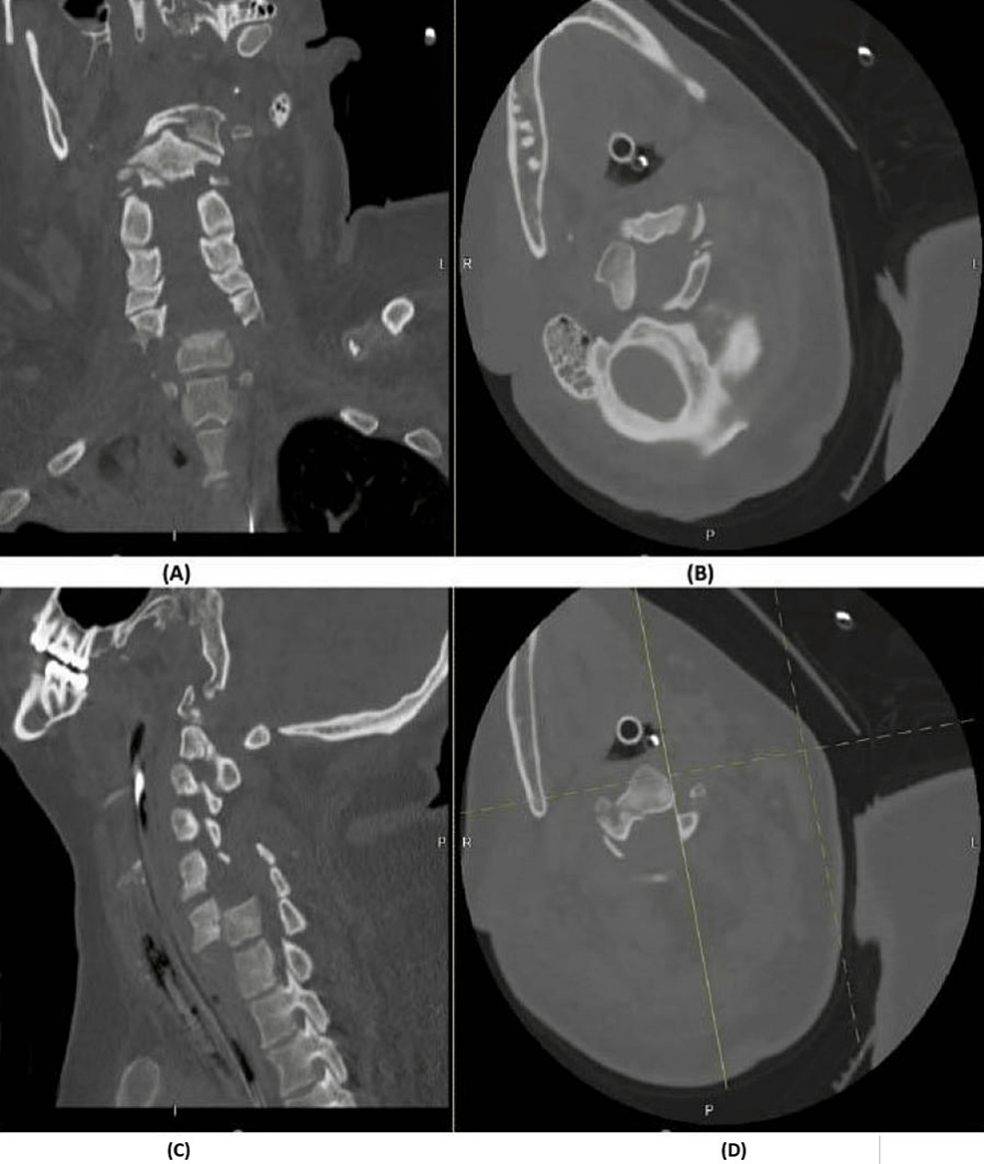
CT scan depicting C2 fracture (A,C) Cervical spine CT scan sagittal and coronal cuts demonstrating a fracture dissecting through the base of C2 and pars interarticularis and bilateral transverse foramina of C2. (B,D) Axial cervical spine CT cuts of the corresponding level of C1–C2 vertebral body.
